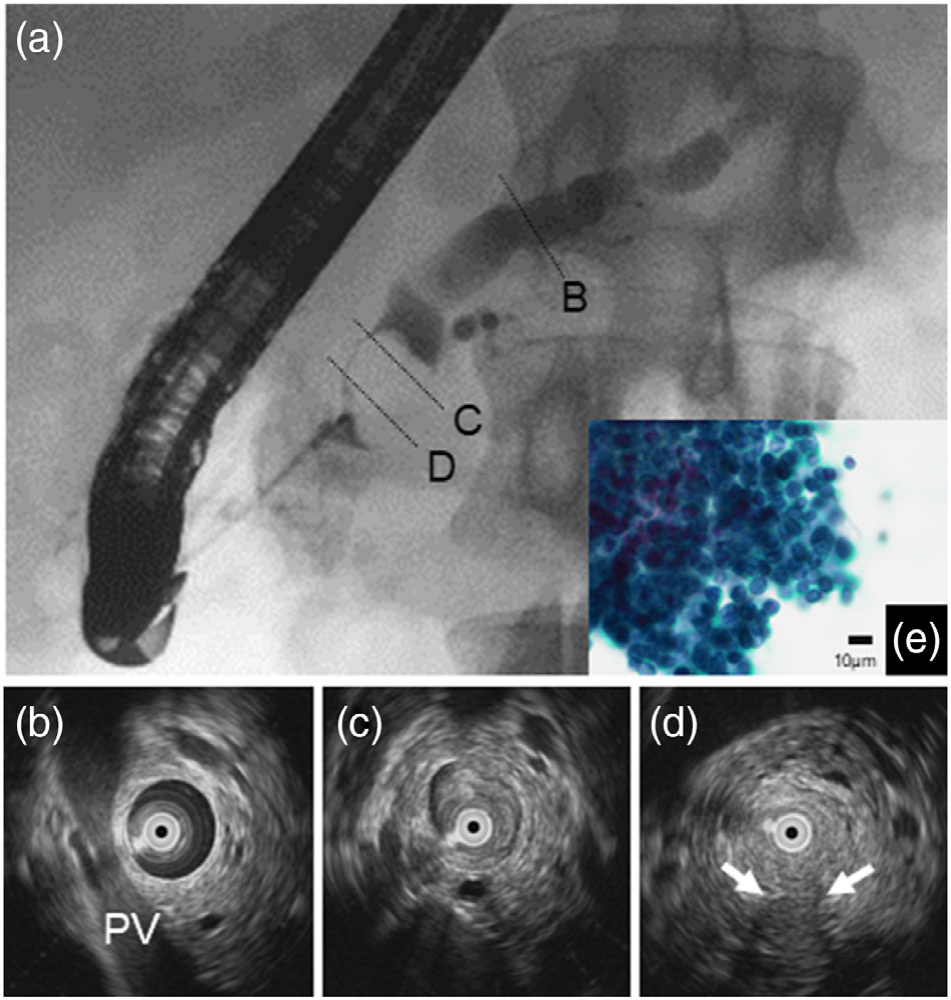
(a) Endoscopic retrograde pancreatography showing a defect with a smooth surface in the main pancreatic duct (MPD) of the head of the pancreas. (b–d) Intraductal ultrasonography showing an isoechoic mass located predominantly in the MPD and partial infiltration into the pancreatic parenchyma (arrows). (e) Pancreatic juice cytology showing a superimposed "islet‐like" conglomerate composed of small cells, which was atypical for a normal pancreatic ductal carcinoma. PV: portal vein
